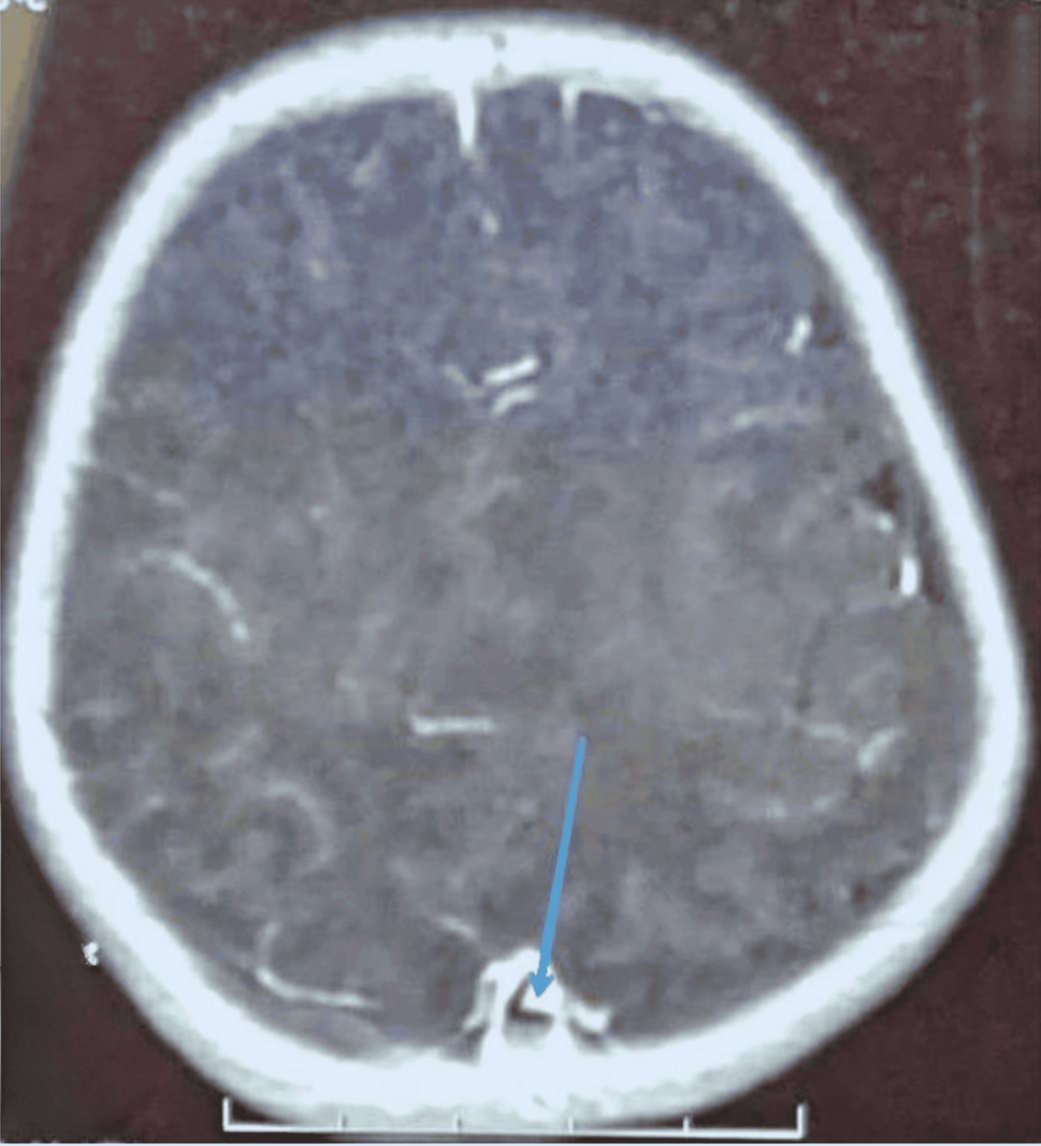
MRI of the patient on day 3 of life in T1 sequence with gadolinium injection, axial view, showing thrombosis in the superior sagittal sinus (blue arrow) MRI: magnetic resonance imaging
